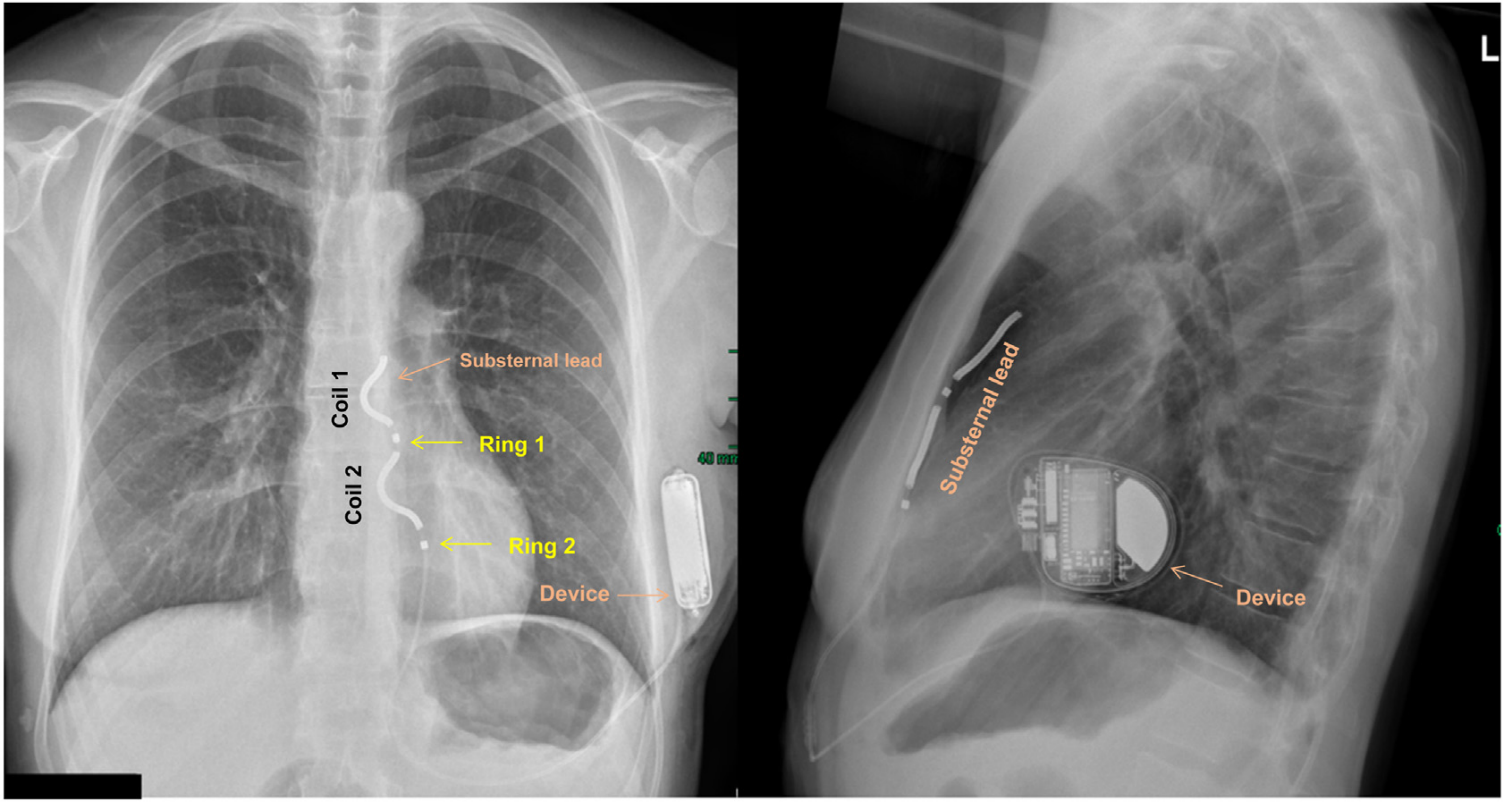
Chest radiograph in posteroanterior (left) and lateral (right) view after successful extravascular implantable cardioverter-defibrillator implantation.
